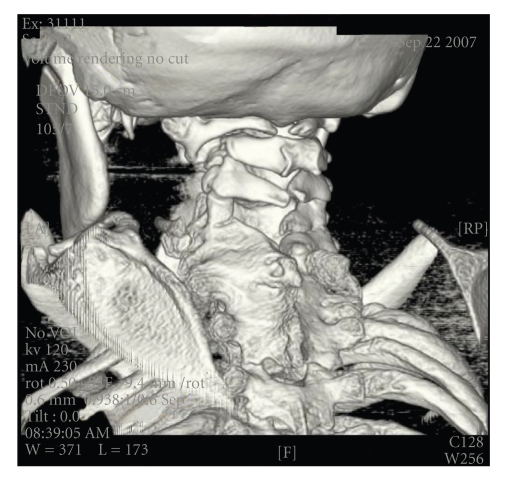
Computed Tomography of the C Spine, with 3D reconstruction, showing Klippel-Feil anomaly comprising of the fusion of multiple cervical and upper thoracic vertebrae.
